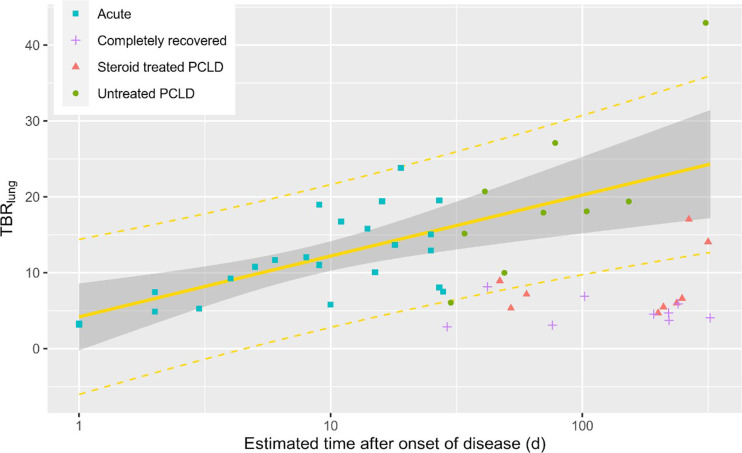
^18^F-FDG uptake (TBR_lung_) against estimated time after onset of disease (on logarithmic scale), with superimposed regression using 23 acute (early and late) patients (*F*_1,23_ = 14.94, *P* < 0.001; Spearman *r*_s_ = 0.595, *P* = 0.003). Steroid treated = at least 10 d of high-dose steroid treatment.
